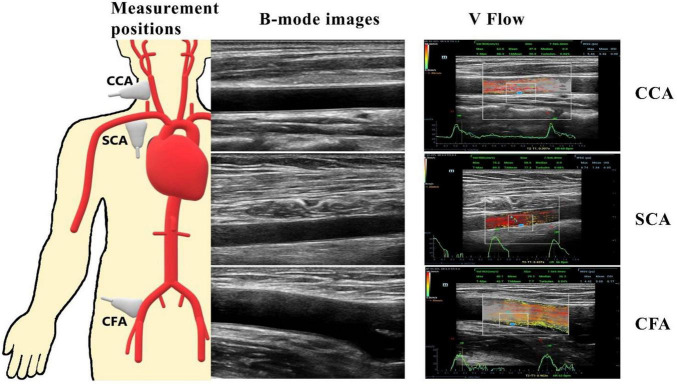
CCA, common carotid artery at 20 to 30 mm of the carotid bifurcation; SCA, subclavian artery at 25 to 35 cm from the start of the SCA; CFA, common femoral artery at 15 to 25 mm of the common femoral artery bifurcation. The pictures on the right represent two-dimensional ultrasound images of the three peripheral arteries and a vector flow imaging technique (V Flow) to measure peripheral arterial hemodynamic parameters, including wall shear stress (WSS) at the place where the blue dot locates and the turbulence index (Turbulen) in the small ROI.
